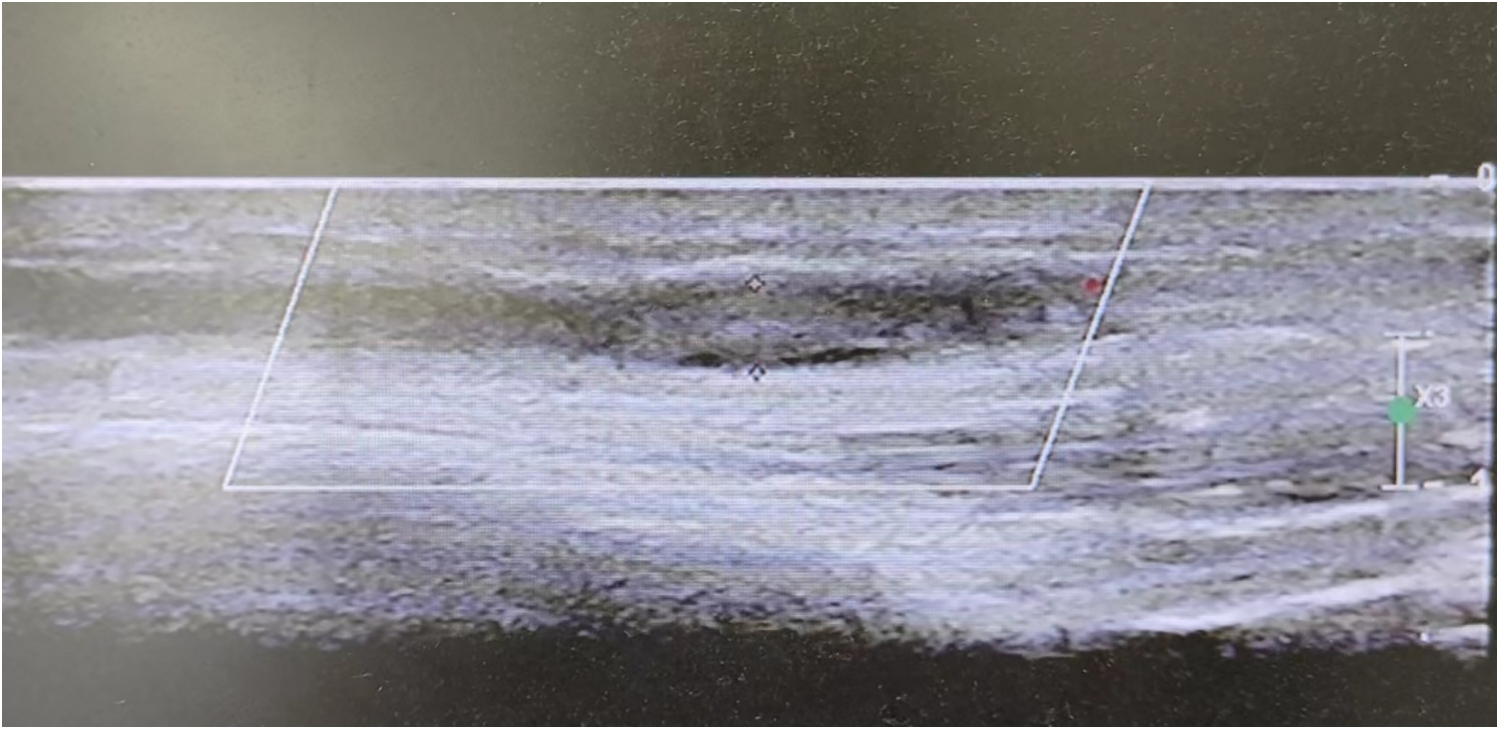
Ultrasound of superficial veins shows the formation of thrombus of patient 1.
